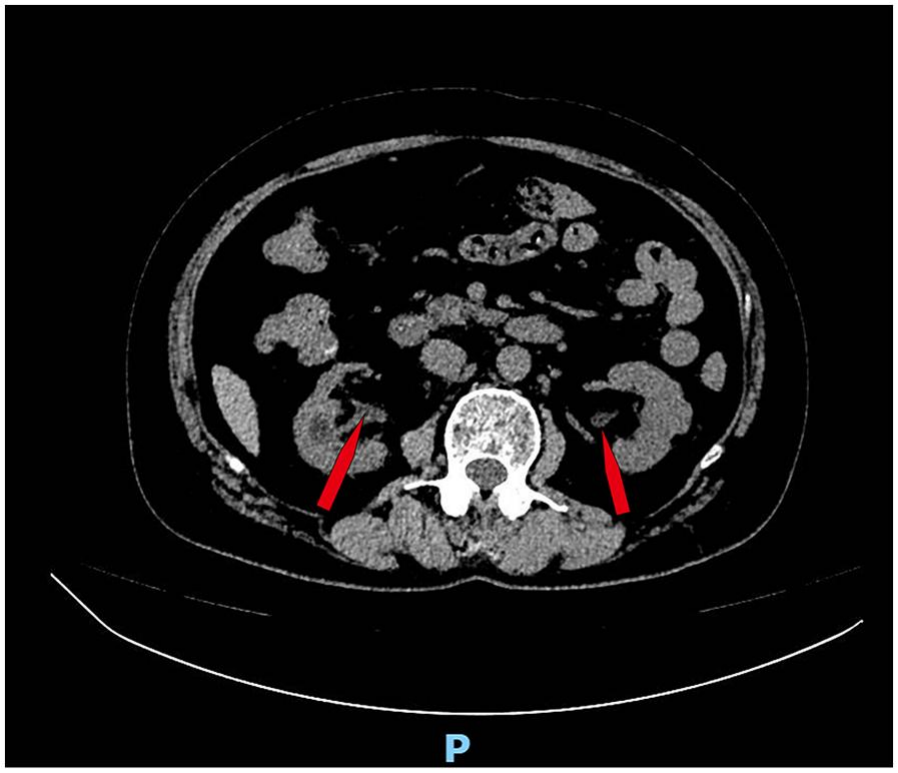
CT scan showed no dilated ureter (Figure 3, red arrow).
